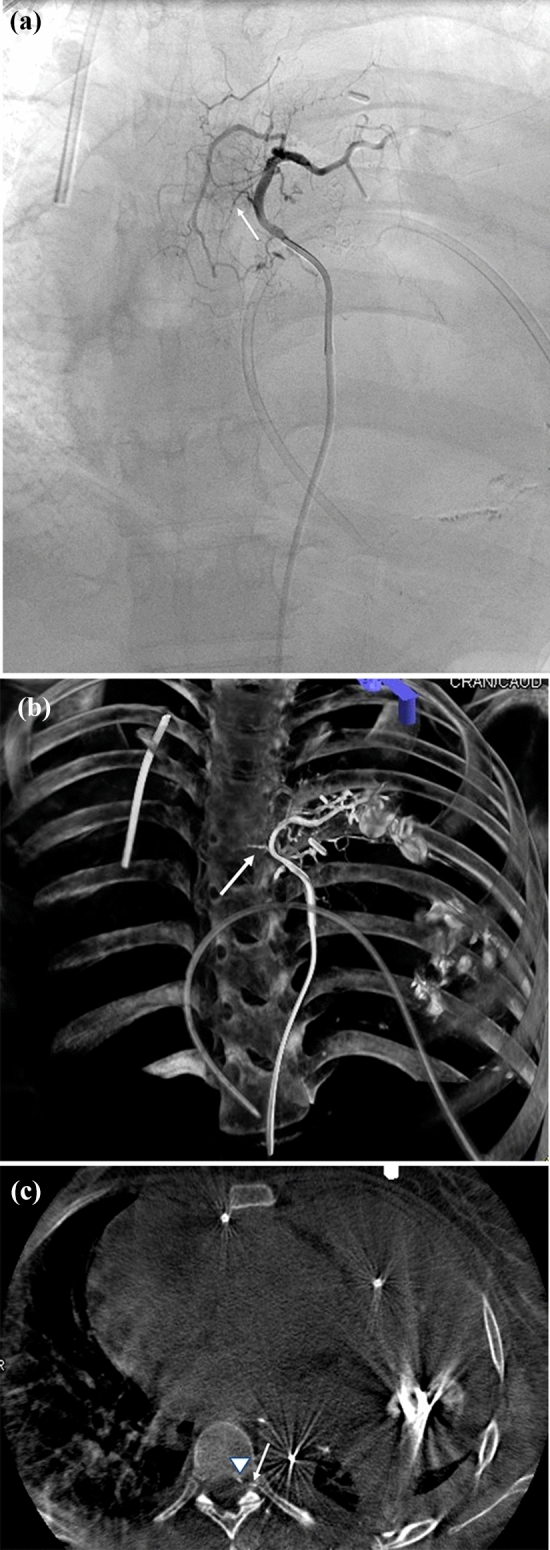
23-y/o female patient with history of hemoptysis from a left thorax synovial cell sarcoma. a DSA coronal image with selective injection of the left bronchial artery with visualization of the minute spinal artery branch seen only in retrospect (white arrow). b, c Volume-rendered image and axial CBCT demonstrating the small spinal artery branch—traversing the intervertebral foramen (white arrow) into the spinal canal (arrowhead)—which was overlooked at time of procedure
